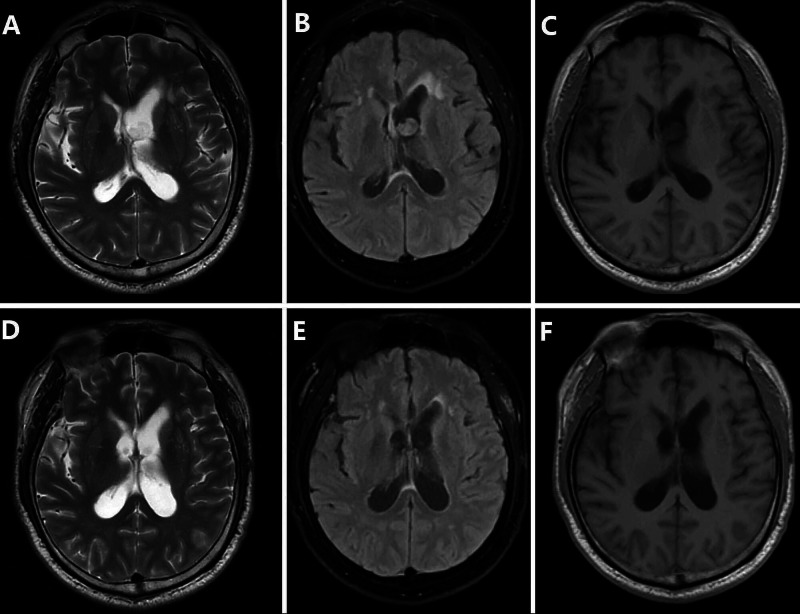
Pre- and post-operation MRI. Head magnetic resonance imaging scan before the operation showing the tumor (A, B, C); brain MRI scan at three months after the operation demonstrating a nearly normal lateral ventricle (D, E, F).
